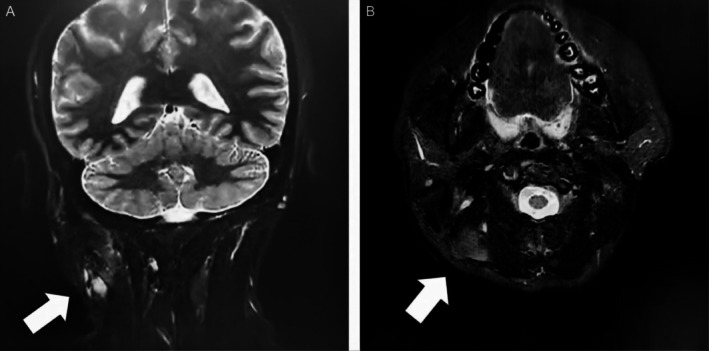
Preoperative Magnetic Resonance Imaging (MRI) assessment. (A) Coronal T2‐weighted fat‐saturated (T2WI Fat‐Sat) sequence shows soft tissue swelling in the right postauricular and mastoid region (white arrow). (B) Axial T2‐weighted fat‐saturated (T2WI Fat‐Sat) sequence demonstrates that, compared to the contralateral side, the right sternocleidomastoid and splenius capitis muscles are enlarged. The mass is closely apposed to the right occipital bone with signs of cortical irregularity, suggesting potential osseous invasion (white arrow).
